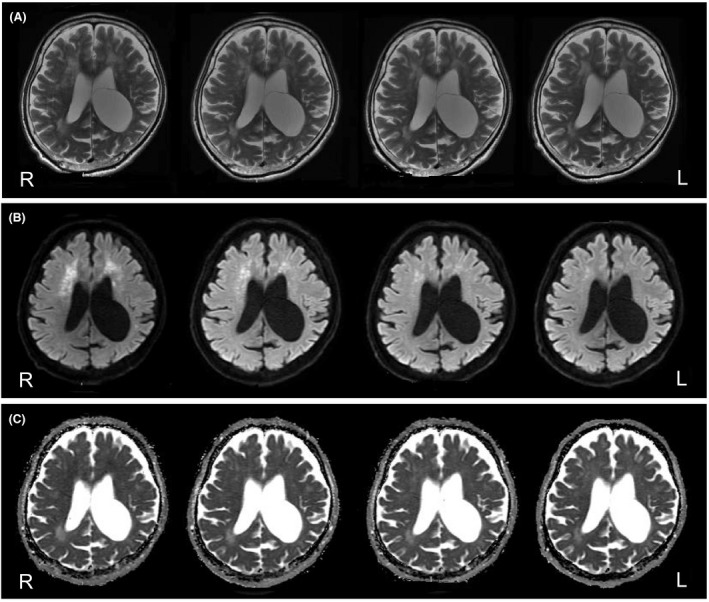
Follow‐up MRIs obtained every 2 months (from left to right). Left periphery images were obtained approximately 2 months after the hypoxic insult. (A) T2 images reveal slight deterioration of the periventricular regions. (B) DWI reveals hyperintensity in the corresponding periventricular regions and that the high signals gradually weakened. (C) The ADC maps reveal slight changes in the corresponding periventricular regions.
